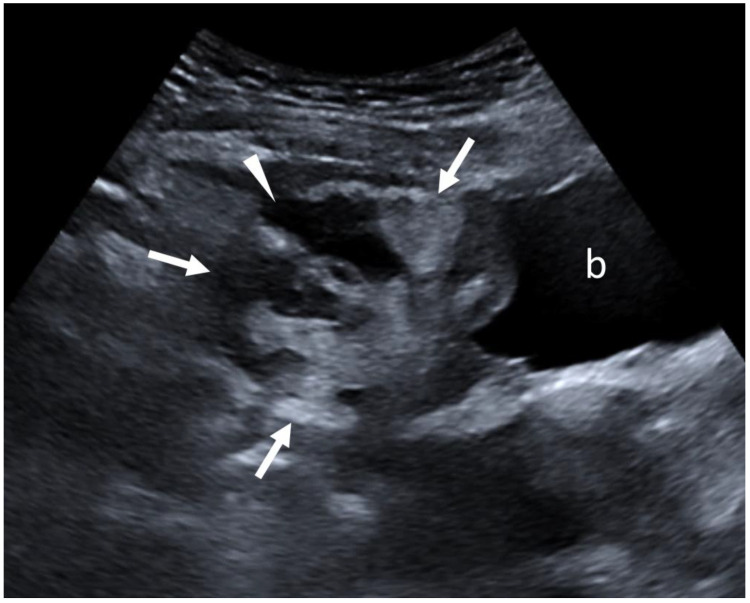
On ultrasound, in a sagittal orientation, the distal part of the greater omentum (white arrows), just above the bladder (b), appeared heterogeneous and was hyperechoic with an anechoic liquid zone corresponding to the abscess in formation (white arrowhead). An ultrasound-guided puncture of the epiploic fluid collection was performed the following day, which confirmed the diagnosis of epiploic abscess with the same bacteria as identified in the endocervical samples. The final diagnosis was therefore that of an epiploic abscess secondary to an upper genital infection with *Parvimonas micra*. The patient received triple antibiotic therapy (ceftriaxone, doxycycline, and metronidazole) for 15 days and was discharged after 3 days in the hospital and went home. Biological inflammatory syndrome disappeared in three days, and a follow-up ultrasound at one month showed complete regression of the greater omentum fluid collection. Omental abscesses can be primary [1] or, most commonly, secondary to spontaneous or post-operative infarction [2,3]. Omental infarction is usually due to venous insufficiency secondary to trauma, abdominal surgery, or venous thrombosis of the veins of the greater omentum [4,5]. Reactive involvement of the greater omentum has already been described in the context of severe pelvic inflammatory disease with tuboovarian abscess, which acts as an internal barrier to contain the infectious process in the pelvis in case of peritonitis [6]. Although MRI is the most accurate imaging modality for the diagnosis of pelvic abscesses, particularly those secondary to pelvic inflammatory diseases [7], CT and ultrasound are easily accessible modalities that allow for the suspicion of the diagnosis in this clinical case. This observation shows the possible occurrence of a greater omentum abscess secondary to a non-severe pelvic inflammatory disease.
